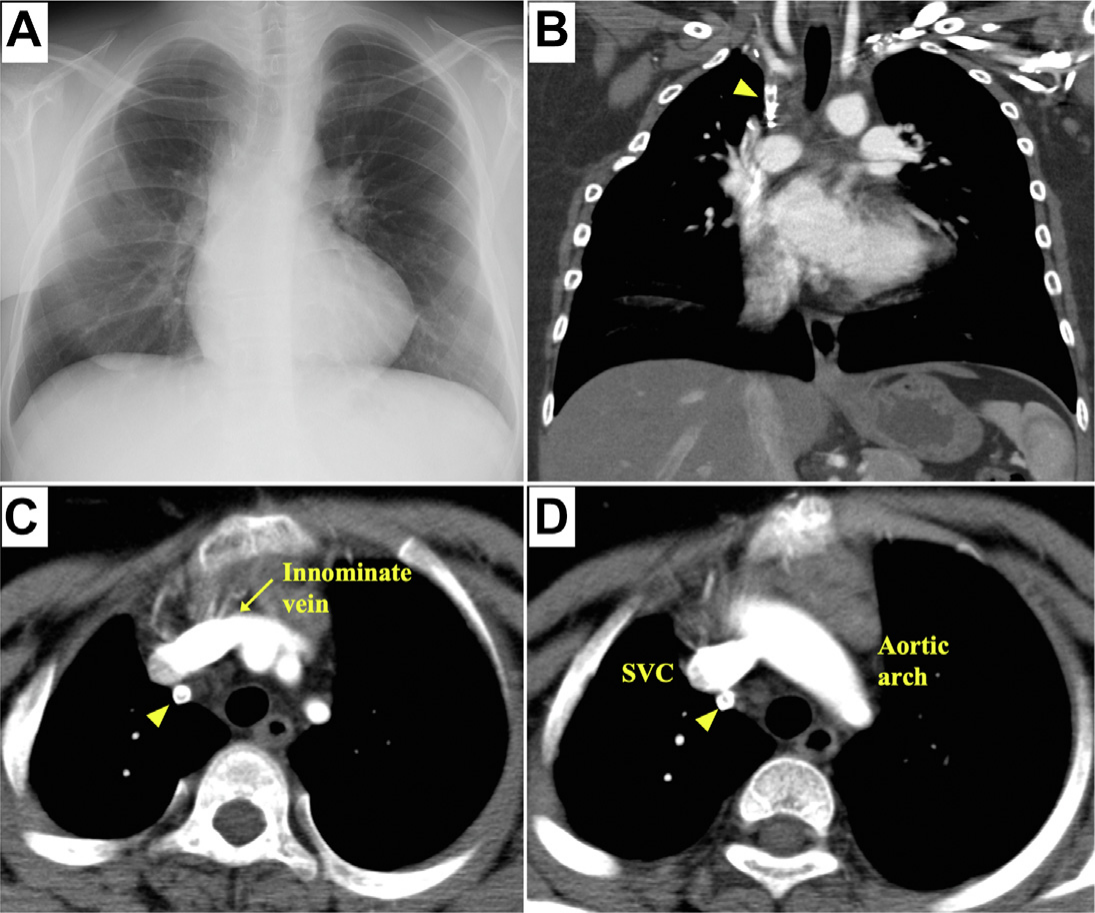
Radiologic images before the current trauma. (A) Chest radiograph 6 months before the injury had no apparent abnormality. (B-D) Chest computed tomography scans at 9 years old. (Arrowhead indicates Blalock-Taussig shunt; SVC, superior vena cava.)
